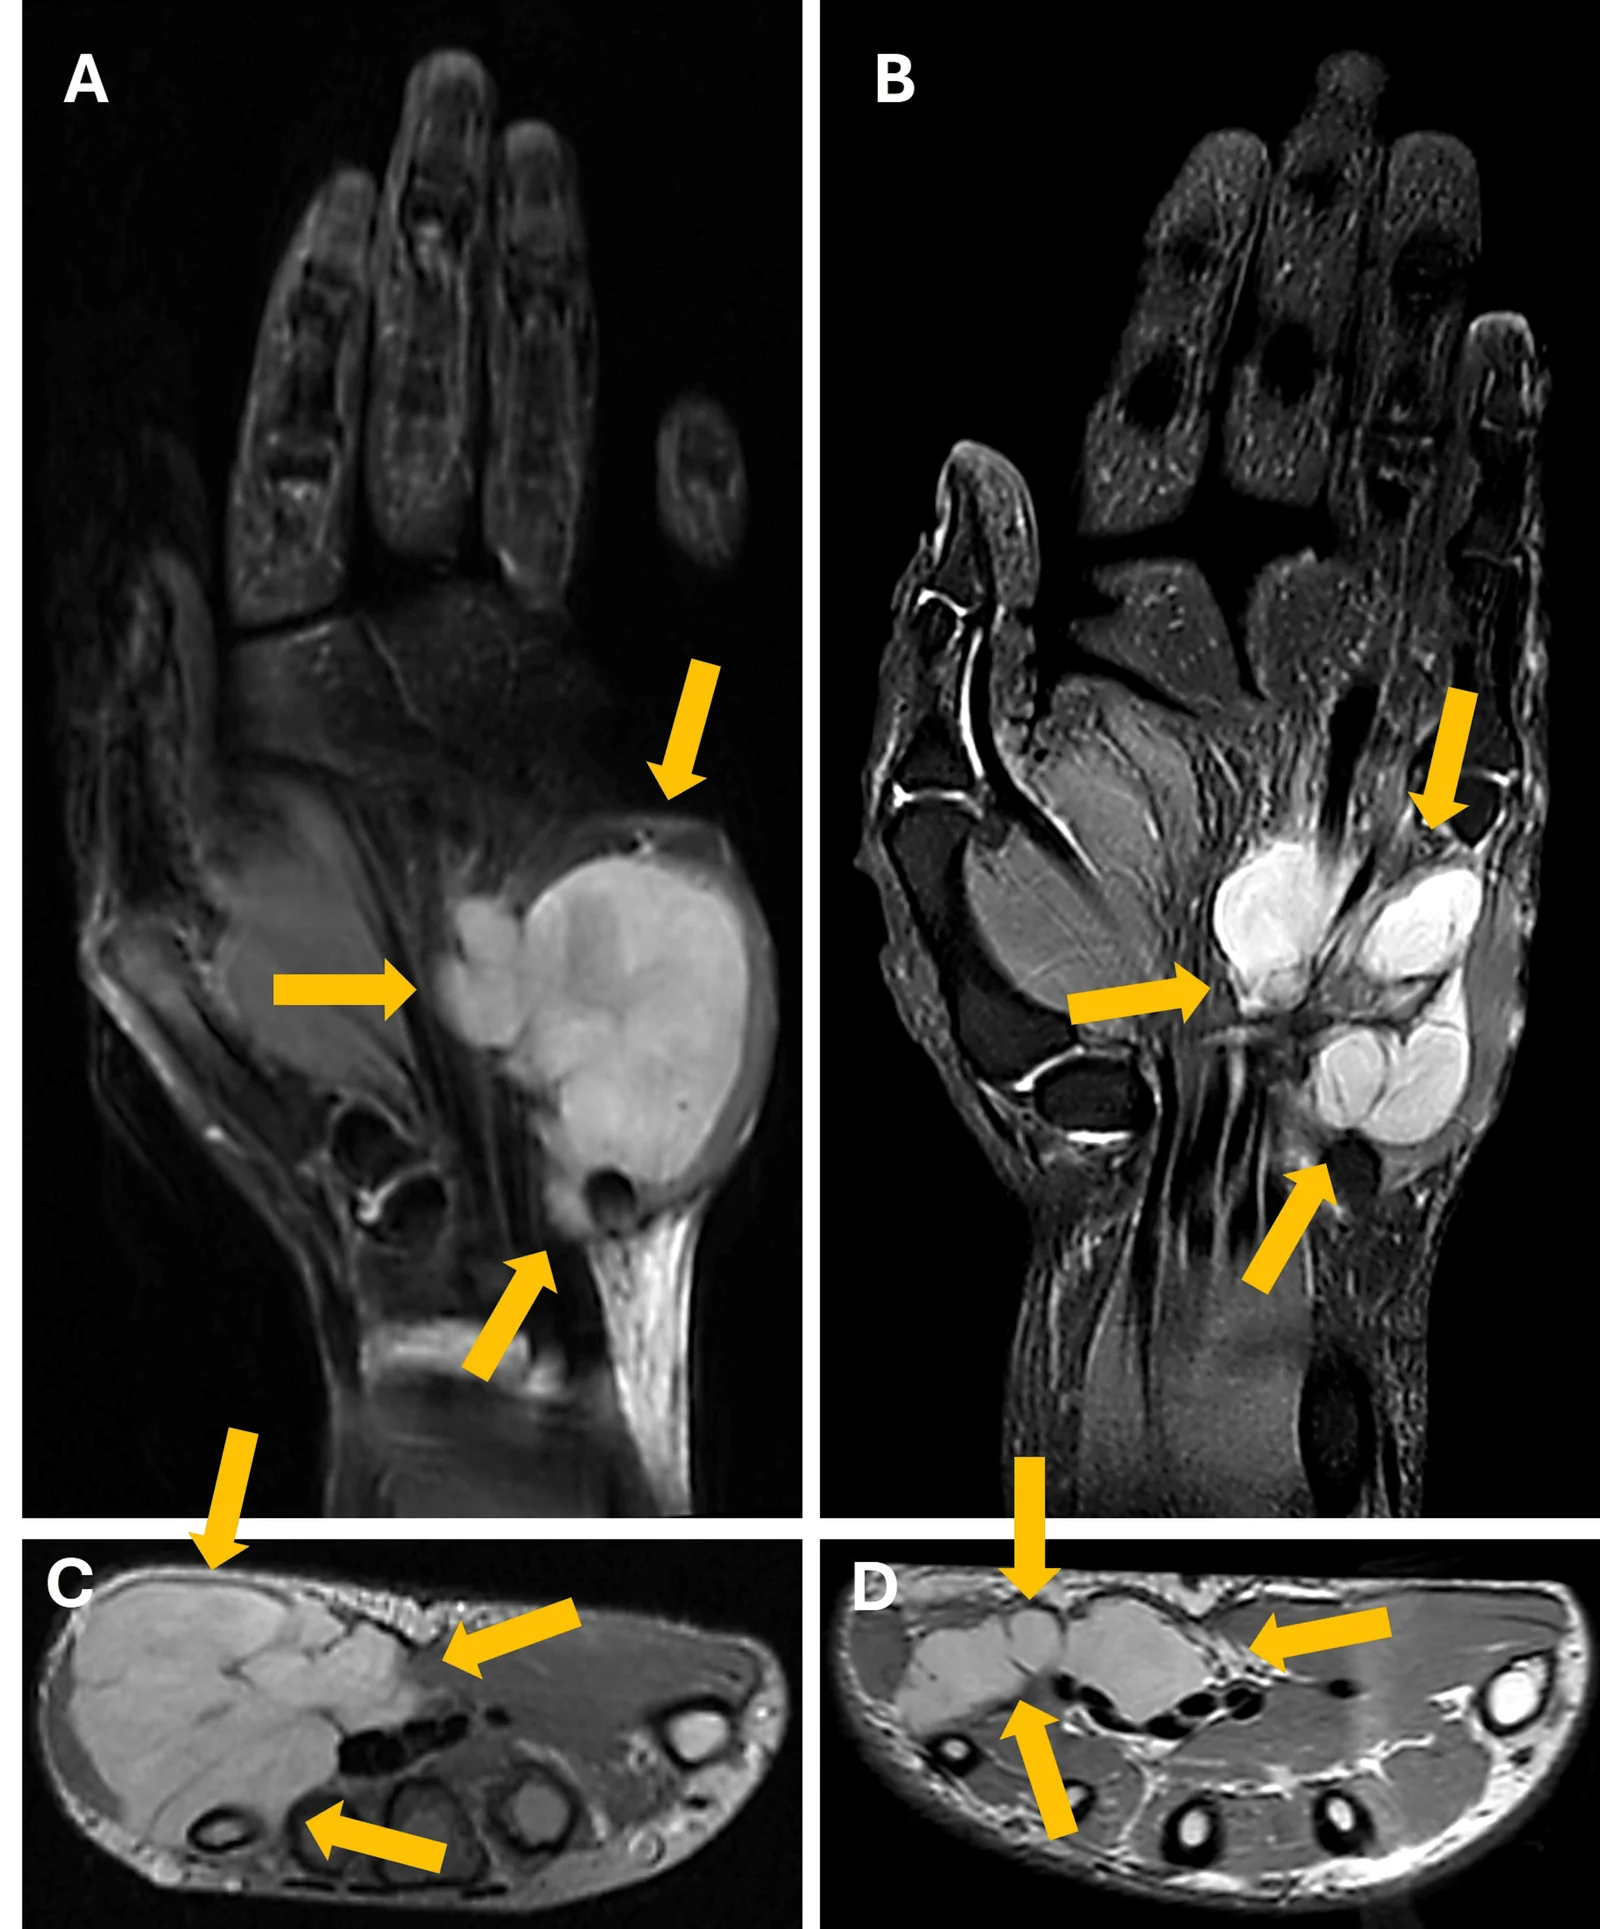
Magnetic resonance imaging of the clear cell sarcoma of the hand Pre-radiotherapy: Multilobulated, predominantly cystic tumor in the hypothenar eminence, with thin hypointense septa, measuring 4.8 x 3.4 x 4.9 cm, extending into the space between the carpal tunnel and the hamate bone in the lateral palmar septum, encompassing the fourth and fifth metacarpals (MTC) in A) coronal and C) axial view. Post-radiotherapy: Tumor with the same characteristics but smaller, measuring 3 x 3.1 x 3.7 cm (previously 1.2 x 3.1 x 4.5 cm), displacing the carpal and cubital tunnel anteriorly, in B) coronal view and D) axial view. The margins of the tumor are marked with orange arrows.
